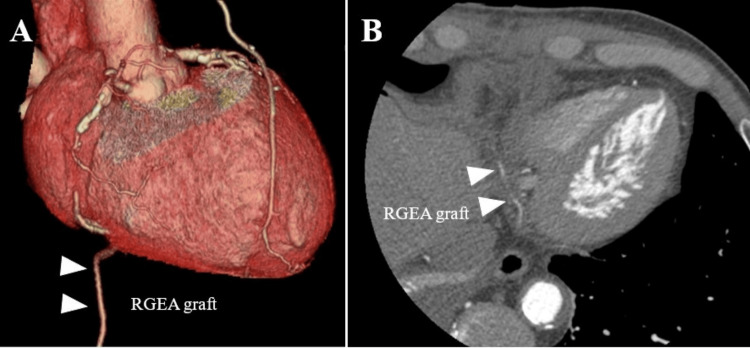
Coronary CT angiography findings before gastrectomy. (A, B) Coronary CT angiography showed that the RGEA graft remained well patent (arrows: RGEA graft). RGEA, right gastroepiploic artery
